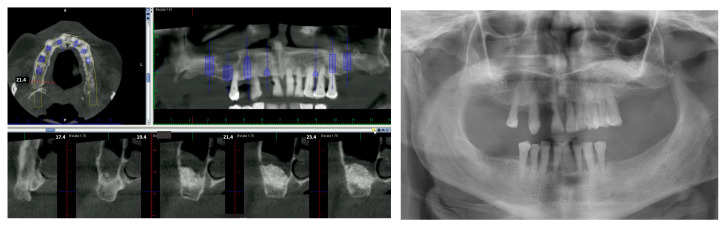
Panoramic radiograph and one of the cuts of planning using CBCT.
